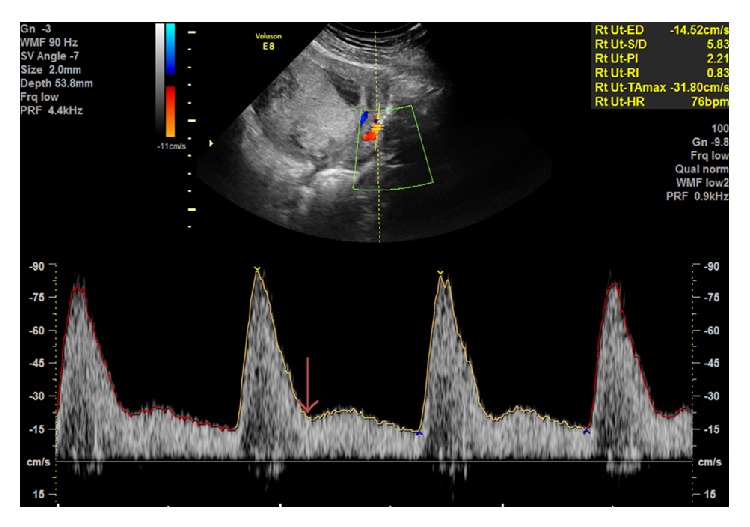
Transabdominal Doppler interrogation of the uterine artery at the level of the internal cervical os. Uterine artery waveform demonstrating raised PI with an early diastolic notch (arrow). Reproduced with permission from Associate Professor F. da Silva Costa.
